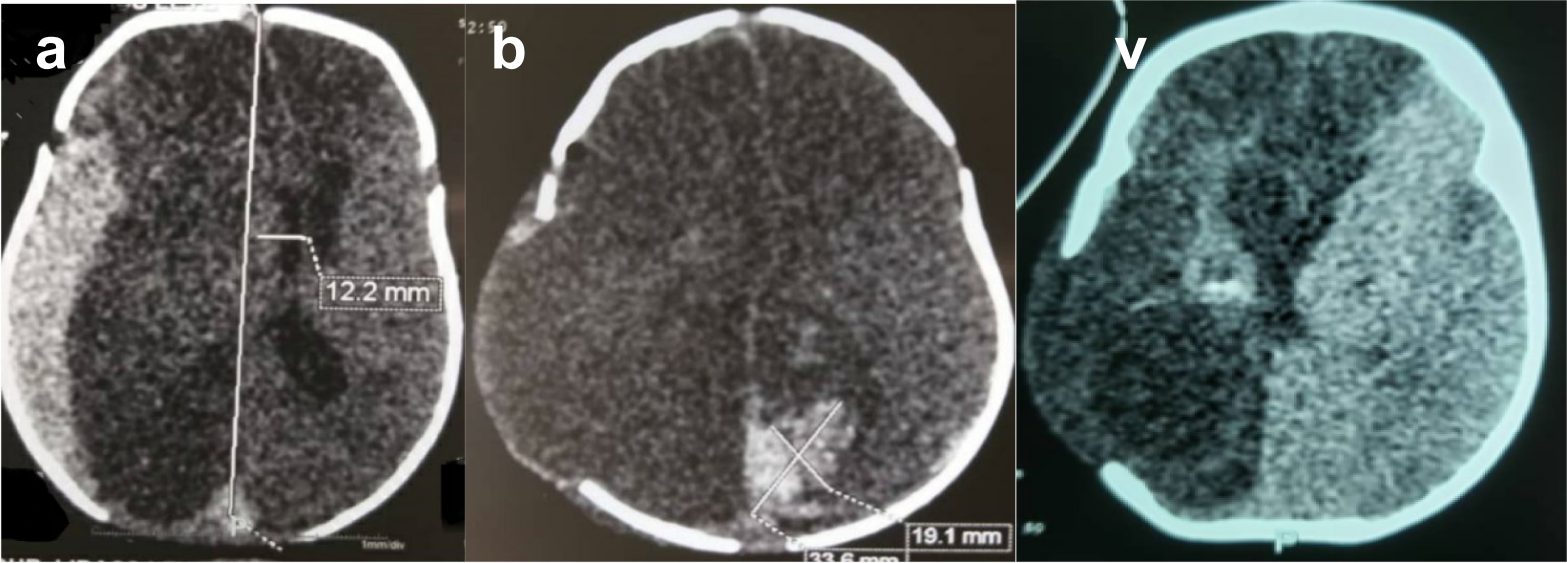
Brain CT scan of a 40-day-old infant showing an acute right parietotemporal subdural hematoma with mass effect (1a). post-decompressive brain CT scan, note the occipital intra-parenchymal hematoma (1b). Control brain CT scan taken 4 months earlier showing sequelae ischemia and the area of craniectomy (1c).
